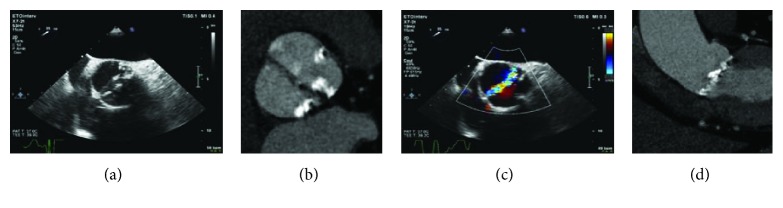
(a, b) Transthoracic echocardiography showing a bicuspid aortic valve. (c, d) Multidetector computed tomography scan revealing a large aortic valve annulus (annular area: 726.9 mm^2^, perimeter: 93.38 mm, long axis: 33.9 mm, and short axis: 26.2 mm) with severe aortic annular calcification.
